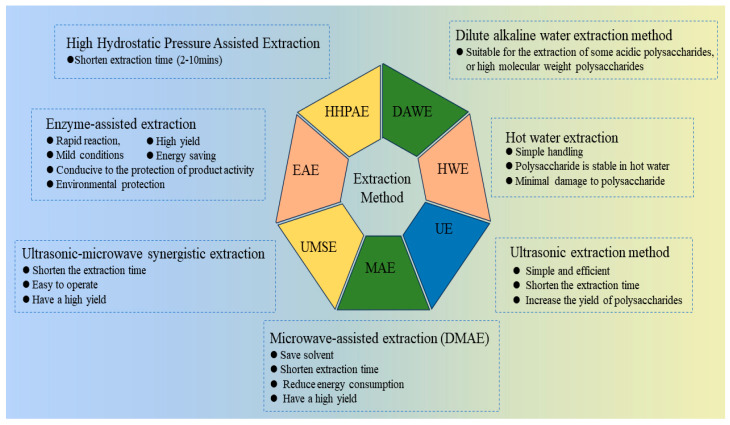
Extraction methods related to *Lycium ruthenicum* Murr. polysaccharide comparisons in terms of advantages. (HWE: Hot water extraction; UEM: Ultrasonic extraction method; MAE: Microwave-assisted extraction; WMSE: Ultrasonic–microwave synergistic extraction; EAE: Enzyme-assisted extraction; HHPAE: High Hydrostatic Pressure Assisted Extraction; DAWE: Dilute alkaline water extraction method).
